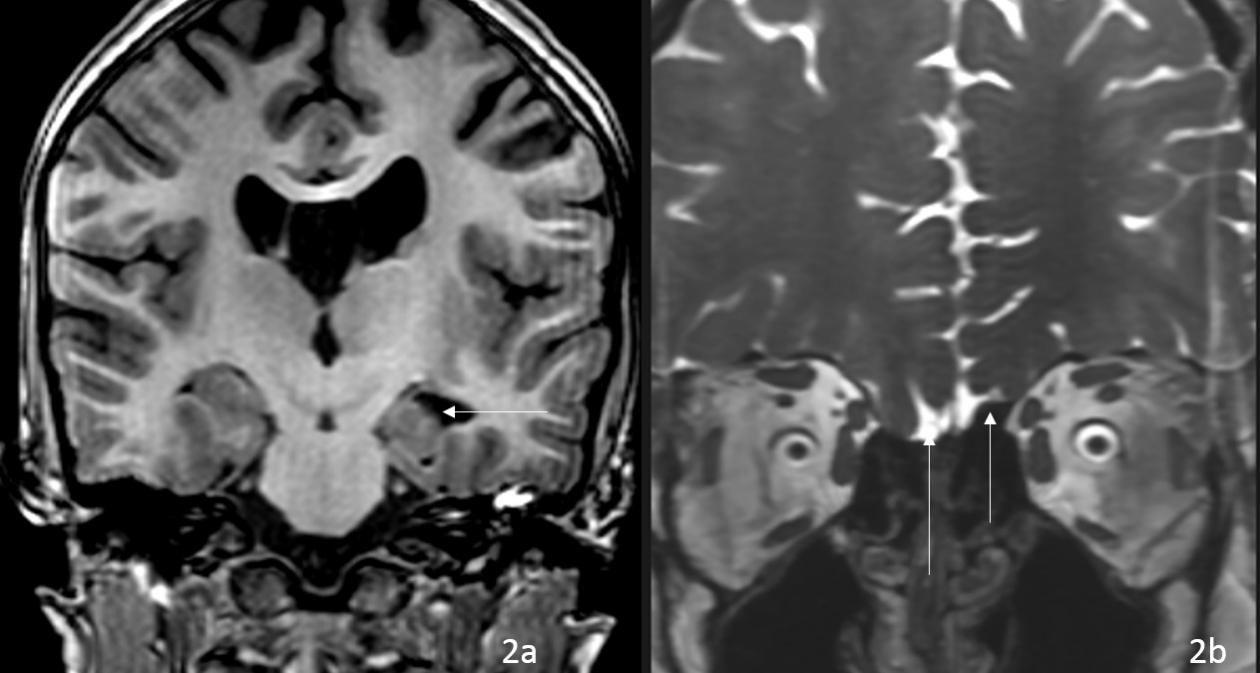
9-year-old female with TMCO1 mutation presenting with epilepsy and MRI demonstrates left hippocampal malrotation (arrow) (2a), absent olfactory bulb (arrow), and hypoplastic bilateral olfactory sulci (2b), bilateral lateral ventriculomegaly and cavum septum pellucidum were present on coronal *T*
